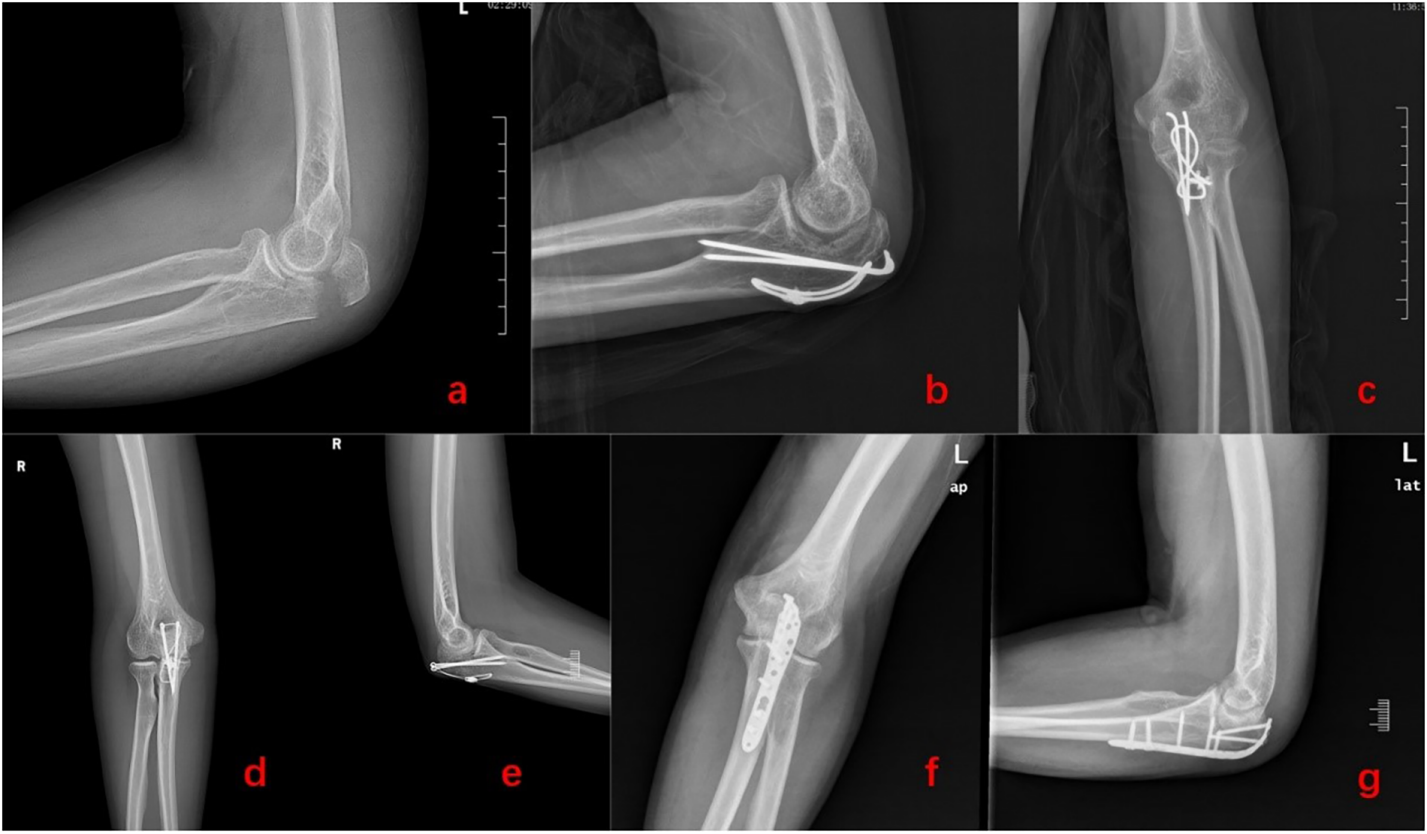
Internal fixation of olecranon fracture, (a) lateral x-ray showing olecranon fracture, (b) lateral x-ray after ordinary Kirschner wire internal fixation, (c) anteroposterior x-ray after ordinary Kirschner wire internal fixation, (d) anteroposterior x-ray after perforated Kirschner wire internal fixation, (e) lateral x-ray after perforated Kirschner wire internal fixation, (f) anteroposterior x-ray after internal fixation of olecranon plate, (g) lateral x-ray after internal fixation of olecranon plate.
